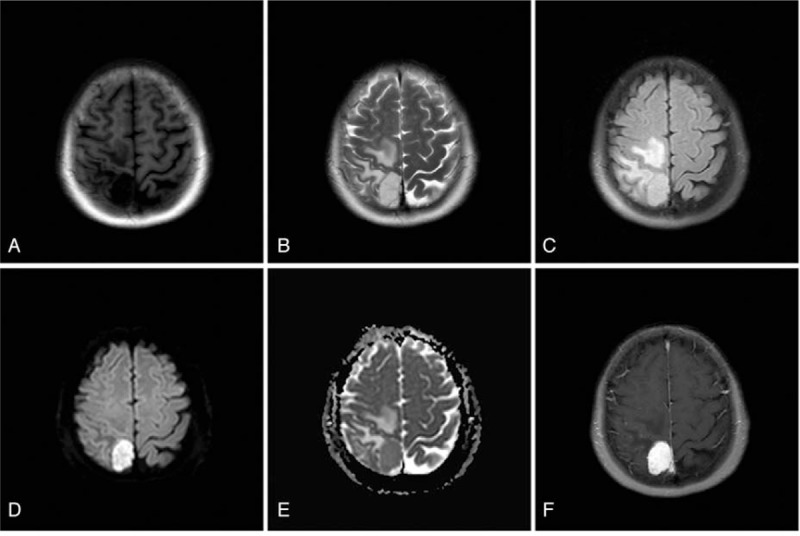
MR imaging features. (A and B) Nodular long T1 long T2 signals are located on the right parietal region. (C–E) The lesion showed high signal on FLAIR (C) and DWI (D) sequence, and low signal intensity on ADC map (E). Obviously homogeneous enhancement was also observed (F).
